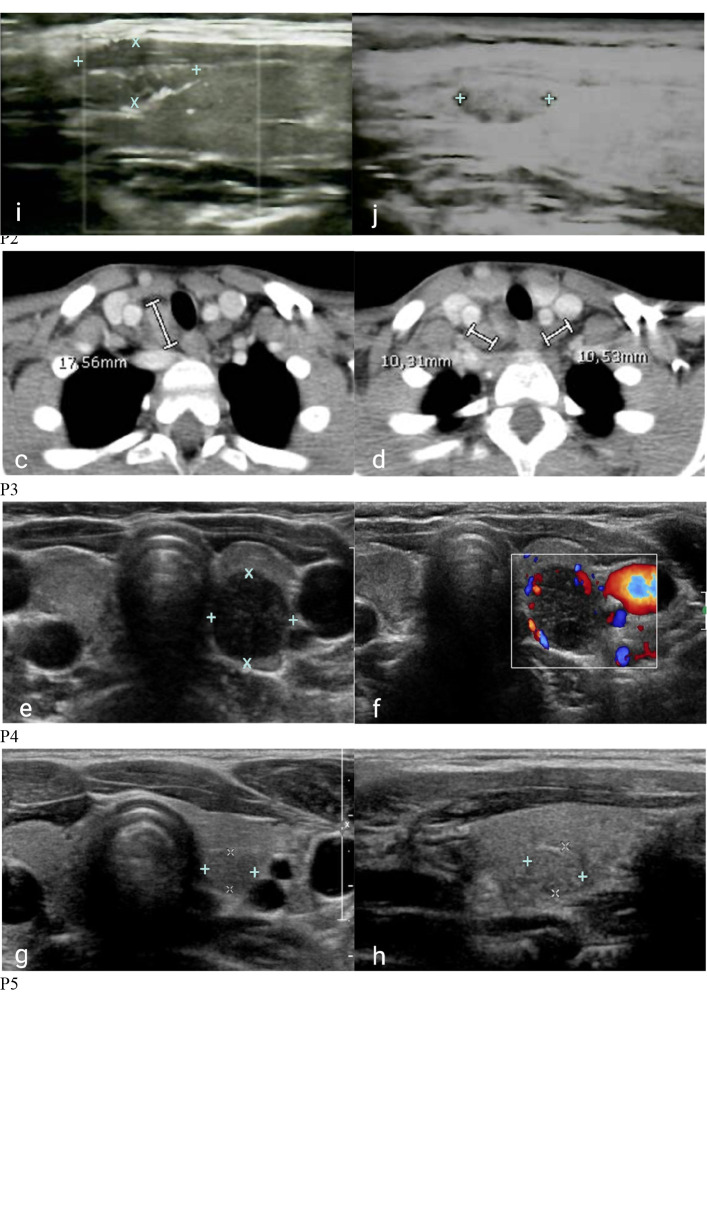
Cervical ultrasonography and computerized tomography of patients with 22q11DS and DiGeorge-like syndrome. P1. Inhomogeneous hypoechoic nodule in the right lower lobe in coronal (A) and sagittal (B) ultrasound projections. P2. Metastatic involvement of right lateral cervical lymph nodes (C) and normal lymph nodes (D) in coronal CT. P3. Inhomogeneous nodule with hypoechogenic areas (E) and peripheral vascular overflow (F) in the left lobe in coronal ultrasound projections. P4. Roundish hypoechoic nodule in the posterior region of the left lobe in close proximity to the carotid artery in coronal (G) and sagittal (H) ultrasound projections. P5. Iso-hypoechoic nodule in the upper third of the right lobe with blurred margins, and intra- and perilesional vascularity in sagittal ultrasound projections in sagittal ultrasound projection: first identification (I) and 1-year follow-up (J).
